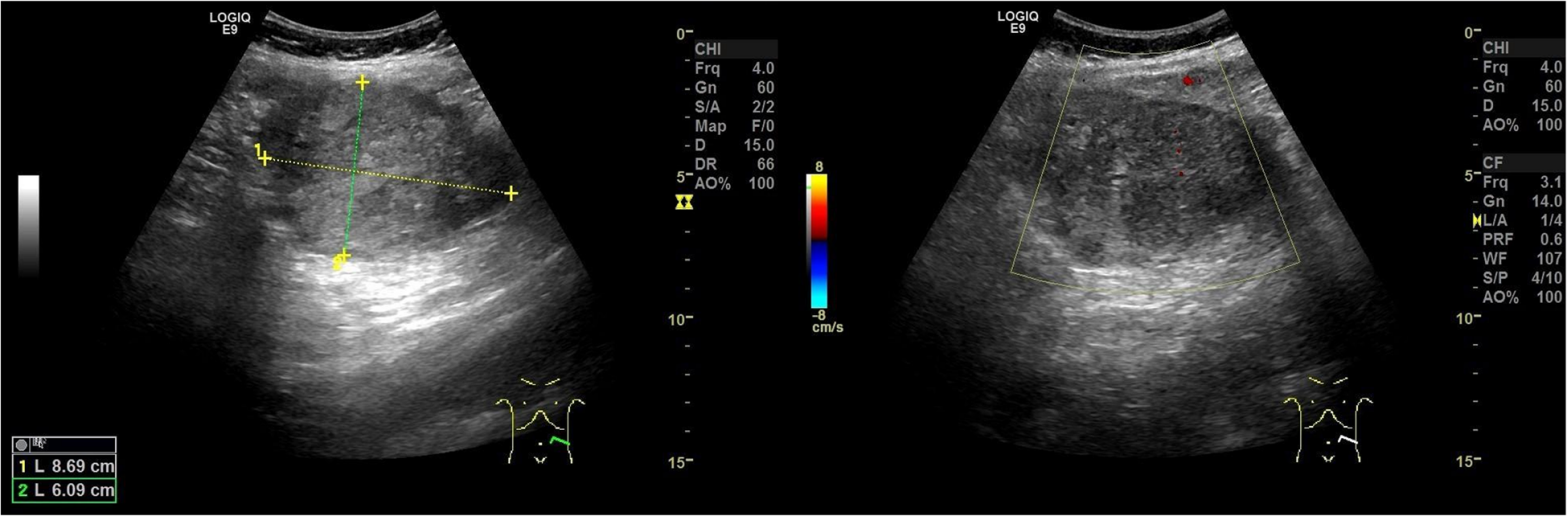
Ultrasound showed a heterogeneously hypoechoic mass in the left abdomen and Doppler revealed a lack of perfusion within the mass
